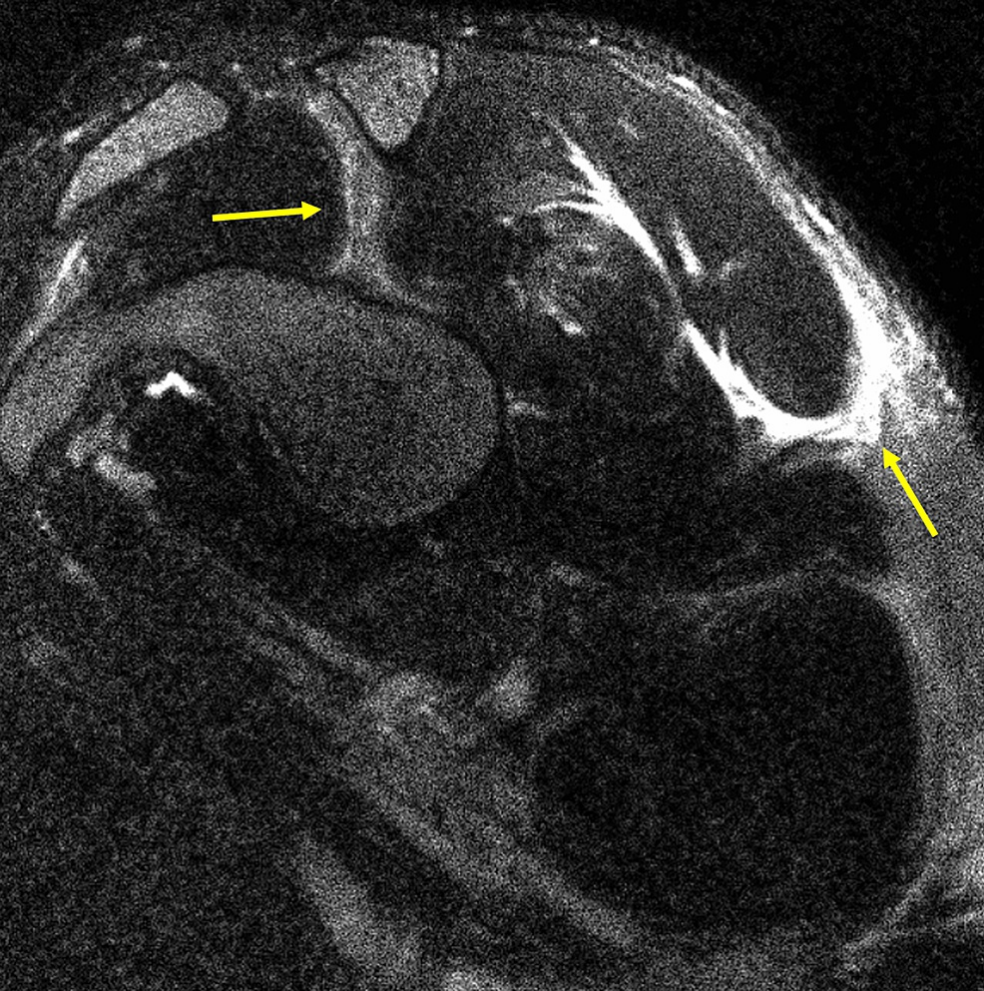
MRI T2-weighted image showing intramuscular edema within the shoulder girdle musculature.
